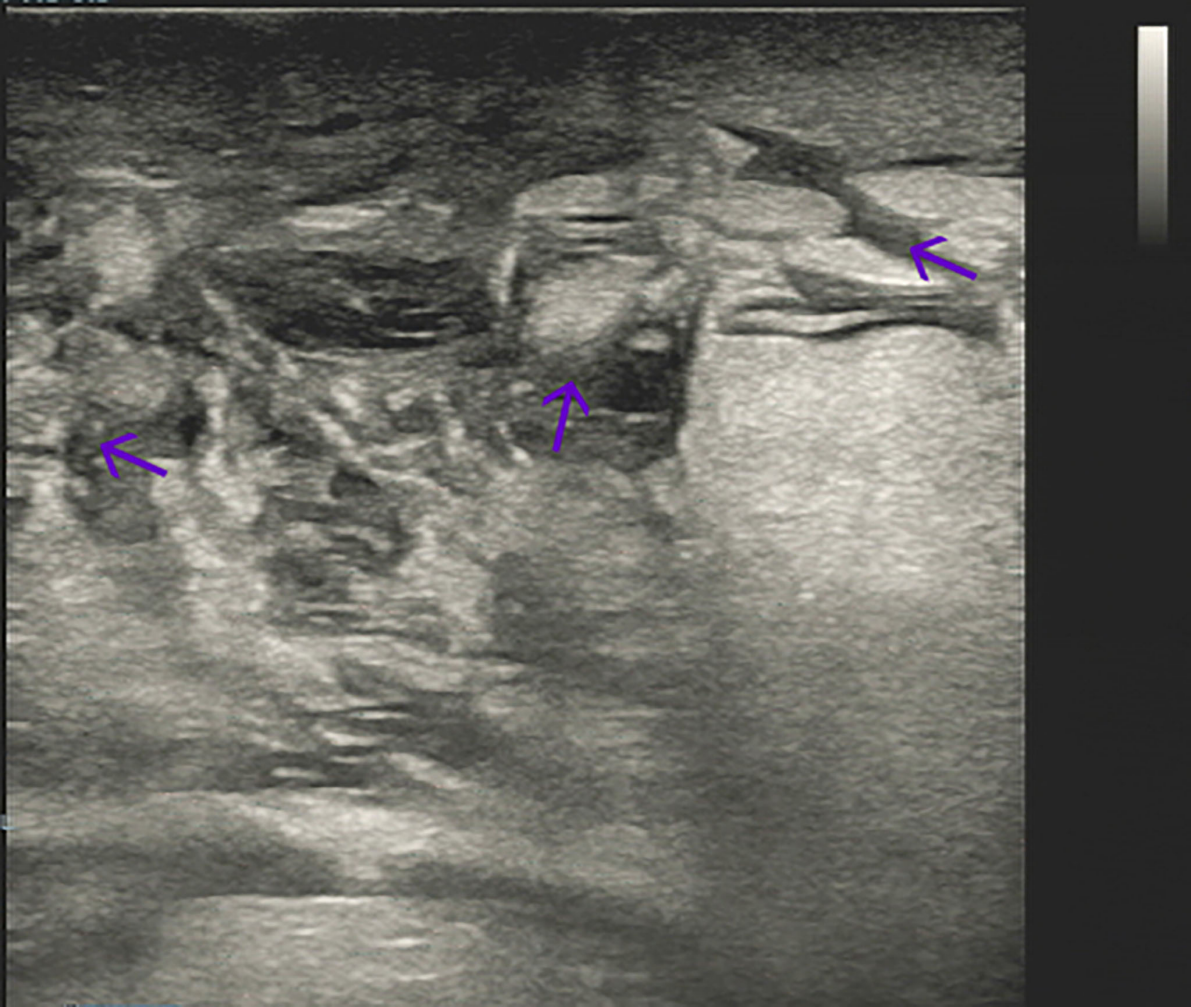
Breast ultrasound showed inflammatory changes in the left breast (the arrow points).
